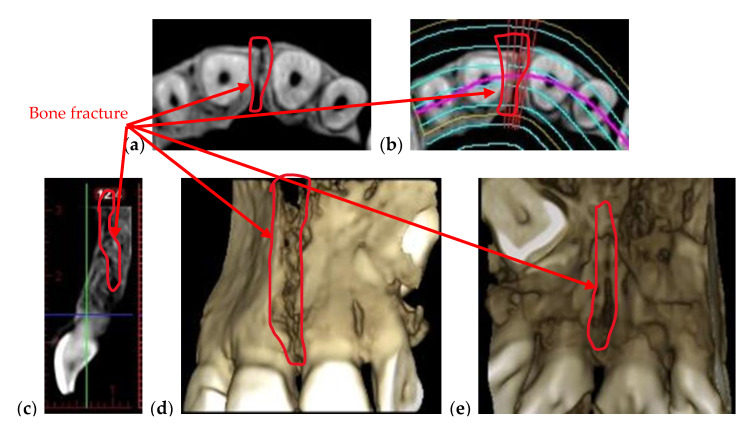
3D CBCT of a fractured maxillary in different views: (a) axial view; (b) axial view indicating the position of the fracture; (c) sagittal view; 3D reconstruction, (d) frontal and (e) posterior view, previously detailed in [28]. Patient V.L., female, age 29 years, diagnosed with a crack in her maxillary bone caused by a head trauma.
